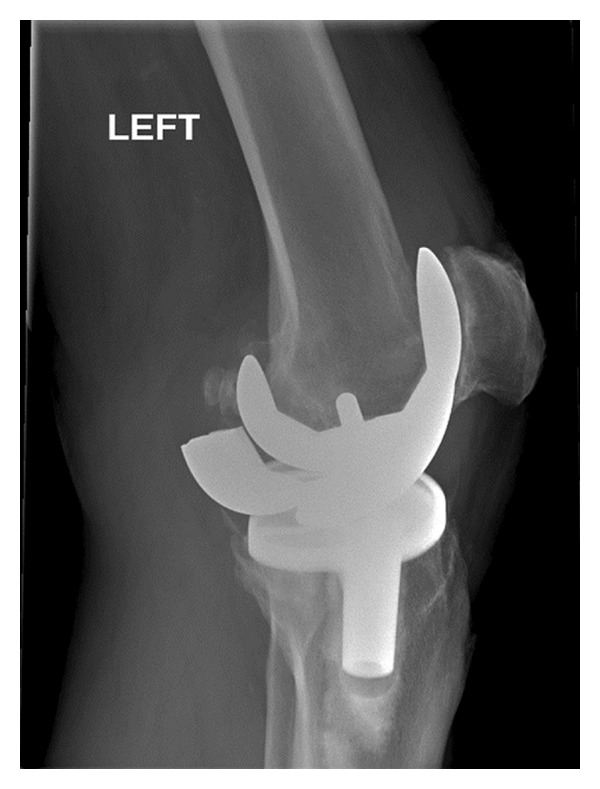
A lateral radiograph of the knee showing the broken prosthesis.
